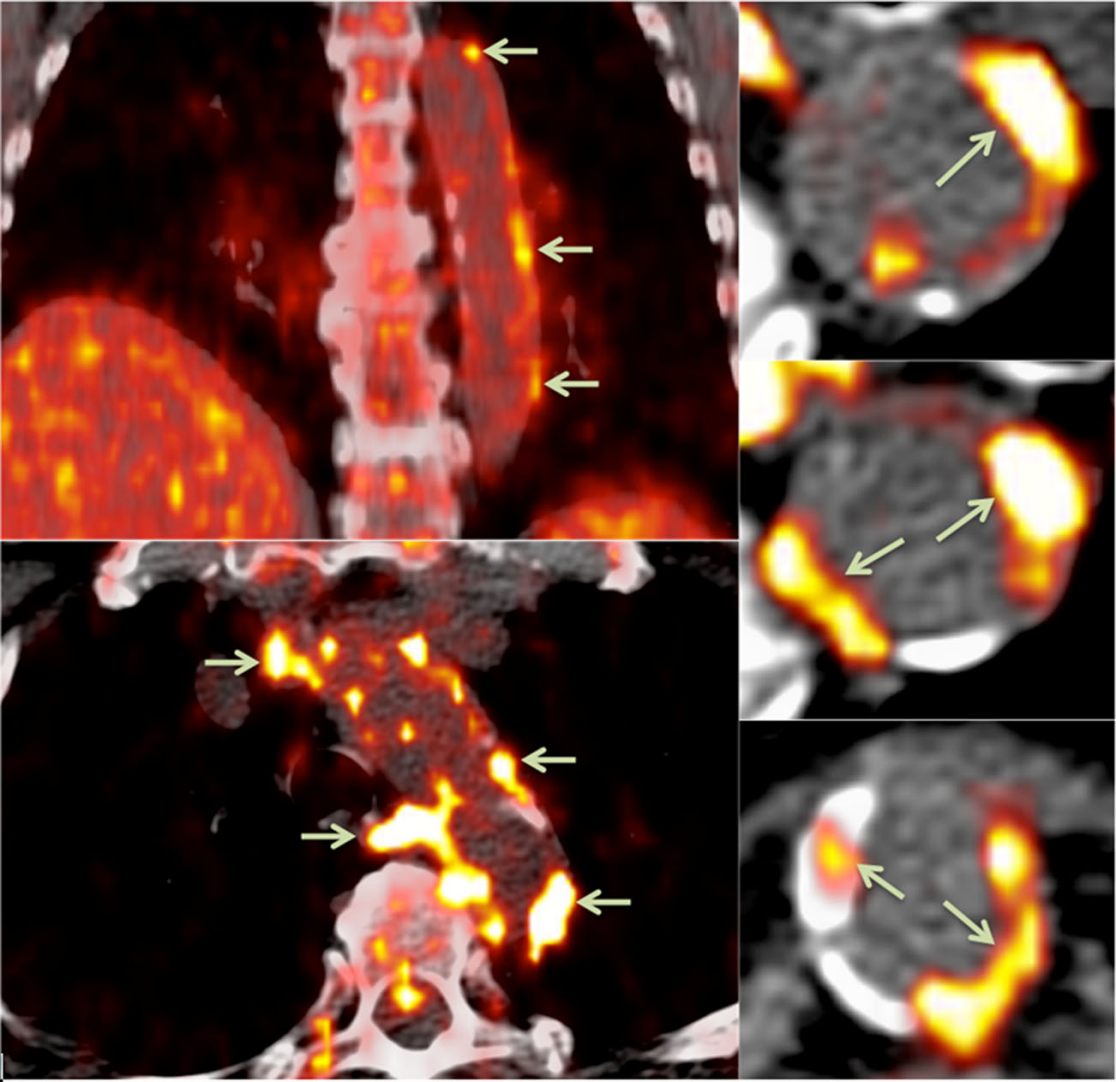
FDG-PET/CT showing areas of focal radiotracer uptake in the wall of the descending aorta (*arrows*)
